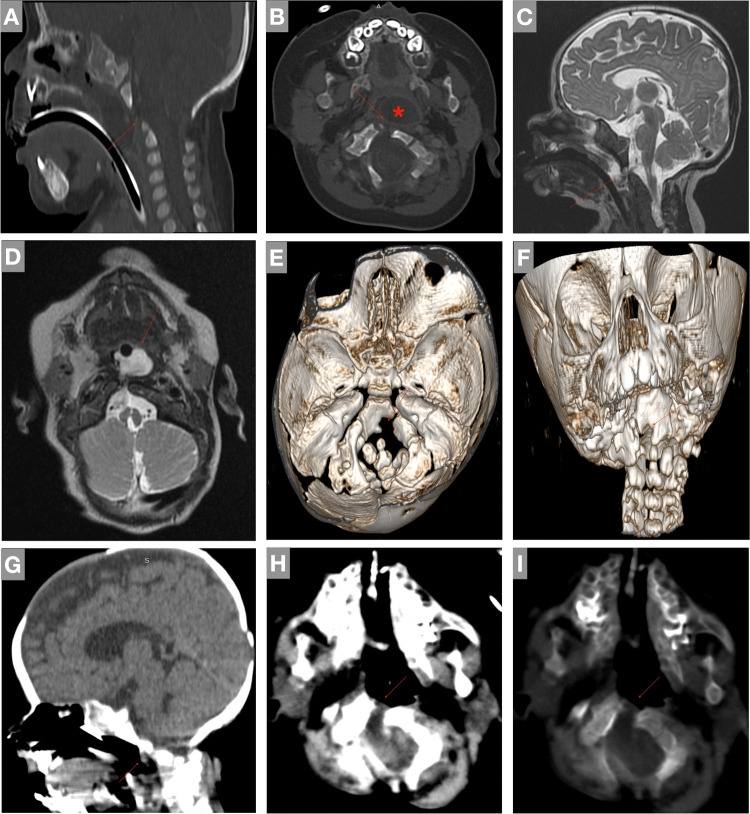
(A-F) Preoperative head CT scan and MRI images and (G-I) postoperative head CT scan. (A, B) Head CT scan—sagittal and axial bone window views showing the clival defect (red arrow) before endoscopic procedure. (C, D) T2-weighted MRI image in sagittal and axial views demonstrating the presence of the cystic lesion before the surgical procedure (red arrow). (E, F) 3D reconstruction of head CT scan showing a congenital hole in the lower third of the clivus located on the right side of the patient (red arrow). (G, H) Soft tissue window—sagittal and axial views demonstrating the absence of the cystic lesion after surgery (red arrow). (I) Bone window—axial view showing the clival defect (red arrow) following the endoscopic procedure, with no evidence of airway obstruction
